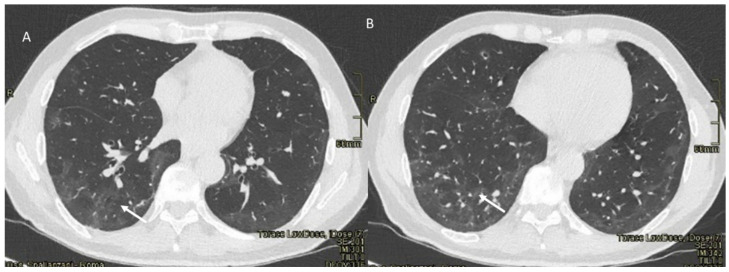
COVID-19 patient. CT (axial plane: (A,B)) shows early stage with GGO (arrow) as main finding.
